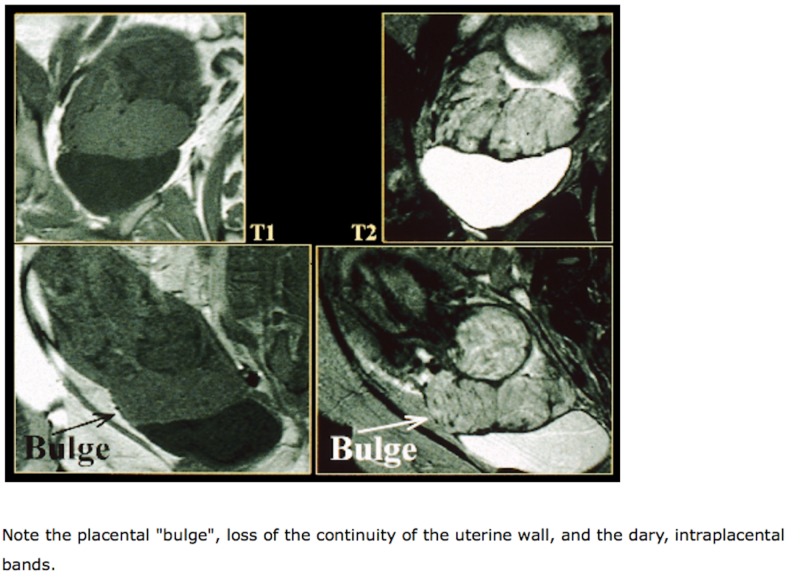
Magnetic resonance imaging suggestive of placenta accreta. Reference [6] This figure was used from UpToDate with consent.
